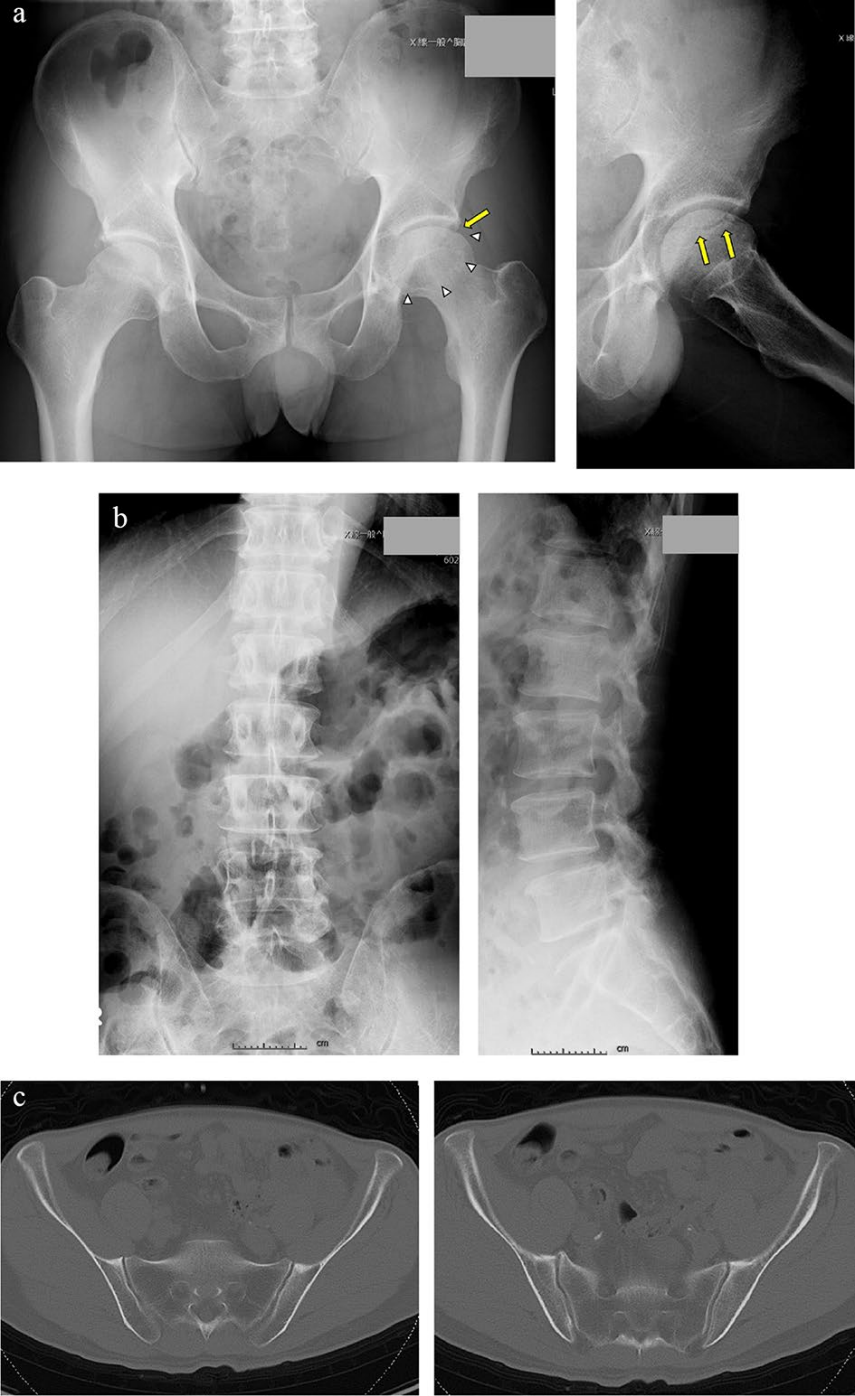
Alcohol-induced ON. (a) The sclerotic boundary was visible (white triangles), with bone collapse on the left femoral head (yellow arrows). (b) X-ray images showed no lumbar spondylolisthesis except for slight scoliosis. (c) CT images showed no abnormalities, including SIJ irregularities. ON: osteonecrosis of the femoral head, SIJ: sacroiliac joint, CT: computed tomography.
